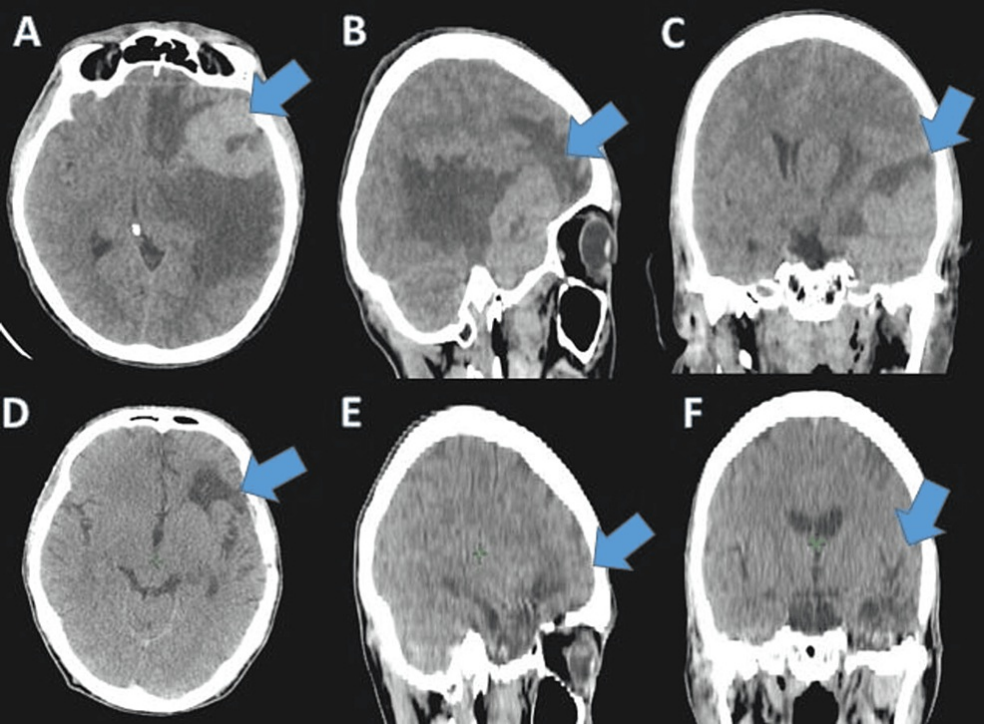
CT in (A, D) axial , (B, E) sagittal, and (C, F) coronal sections showing a left frontotemporal process (A-C) before and (D-F) after chemotherapy.
